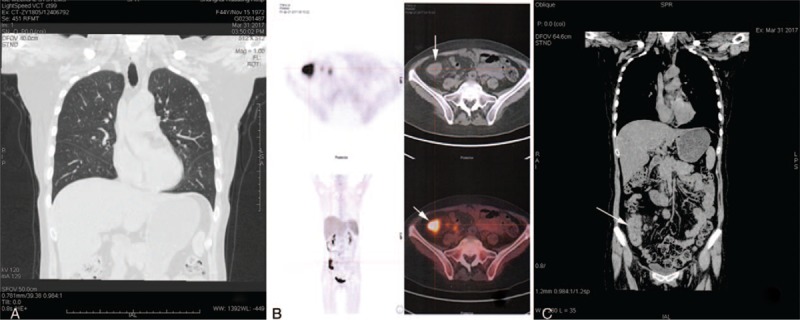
(A) Pulmonary CT examination was normal. (B) PET/CT examination. The ileocecus and ascending colon show increased local sugar metabolism (SUV = 14.1). (C) Abdominal CT examination shows a thickening of the ileocecal wall (white arrow). PET/CT = positron emission tomography/computed tomography); SUV = standard uptake value.
